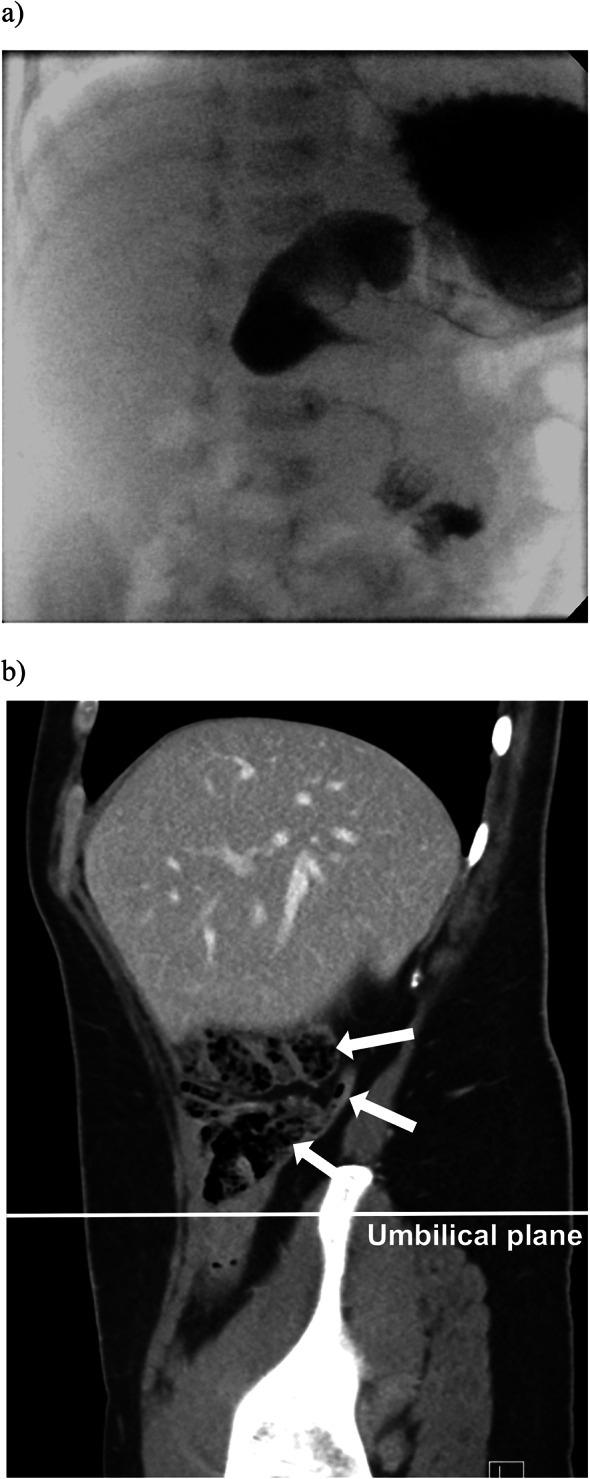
Radiological findings, patient 1 with two mutations in *cis* in the *TTC7A-*gene. a The proband. An upper gastrointestinal study, demonstrating IM with midgut volvulus, here with a “bird’s beak” and corkscrew appearance of the duodenum. b The mother. Computed tomography, a sagittal view demonstrating caecum in the right upper quadrant. Arrows, cranial to caudal direction: the ascending colon, appendix and caecum
